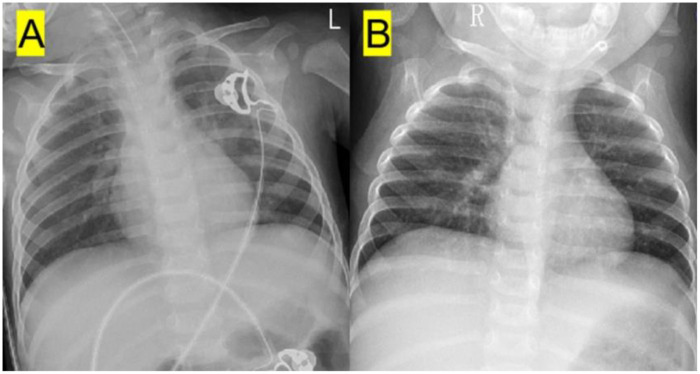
(A) Chest x-ray obtained 9 h after bronchoscopy showing successful left lung re-expansion. (B) Chest x-ray obtained 7 days after bronchoscopy (at discharge) showing resolution of pneumonia.
